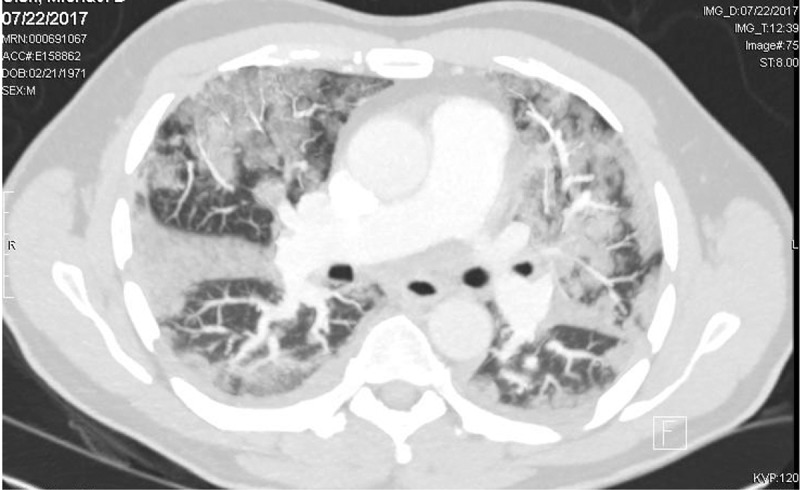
CT Chest shows multifocal patchy airspace opacities and small pleural effusions.
